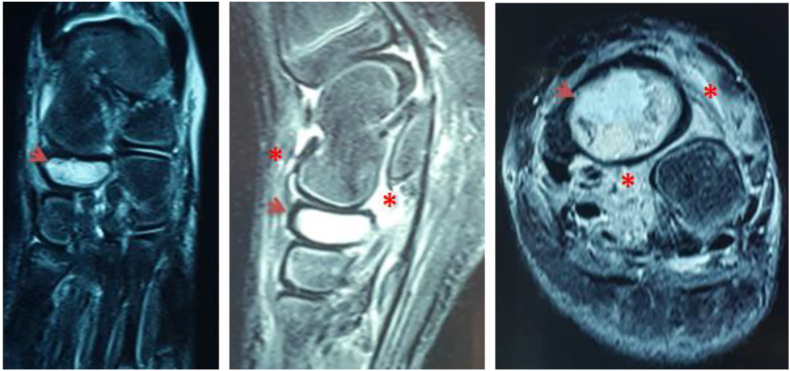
T2-weighted MRI sequences: Intense T2 hypersignal of the spongy bone of the navicular bone (Arrows) on the coronal, sagittal and axial slices associated with an inflammatory reaction in the perinavicular tissue (Asterisk).
